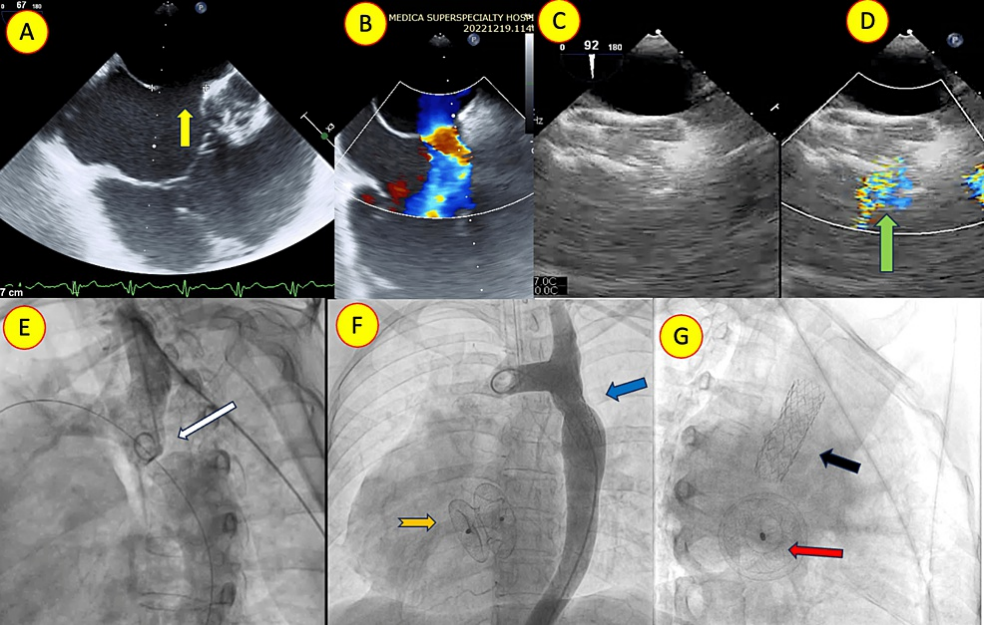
Transesophageal echocardiogram (TEE) with color Doppler (A, B) showing the moderate secundum atrial septal defect (ASD) (yellow arrow) with an absent aortic rim and floppy posterior rim. (G) TEE after device closure with color Doppler (C, D) showing the stable ASD device and fenestration flowing left to right (green arrow). (E) Aortic angiogram in the oblique view showing severe post-subclavian coarctation of the aorta (white arrow). (F) Two-year follow-up aortogram after the ASD device (orange arrow) closure showing good stent flow (blue arrow). (H) Fluoroscopic image showing the fenestration (red arrow) in the oblique view. The good aortic stent position is also seen (black arrow).
